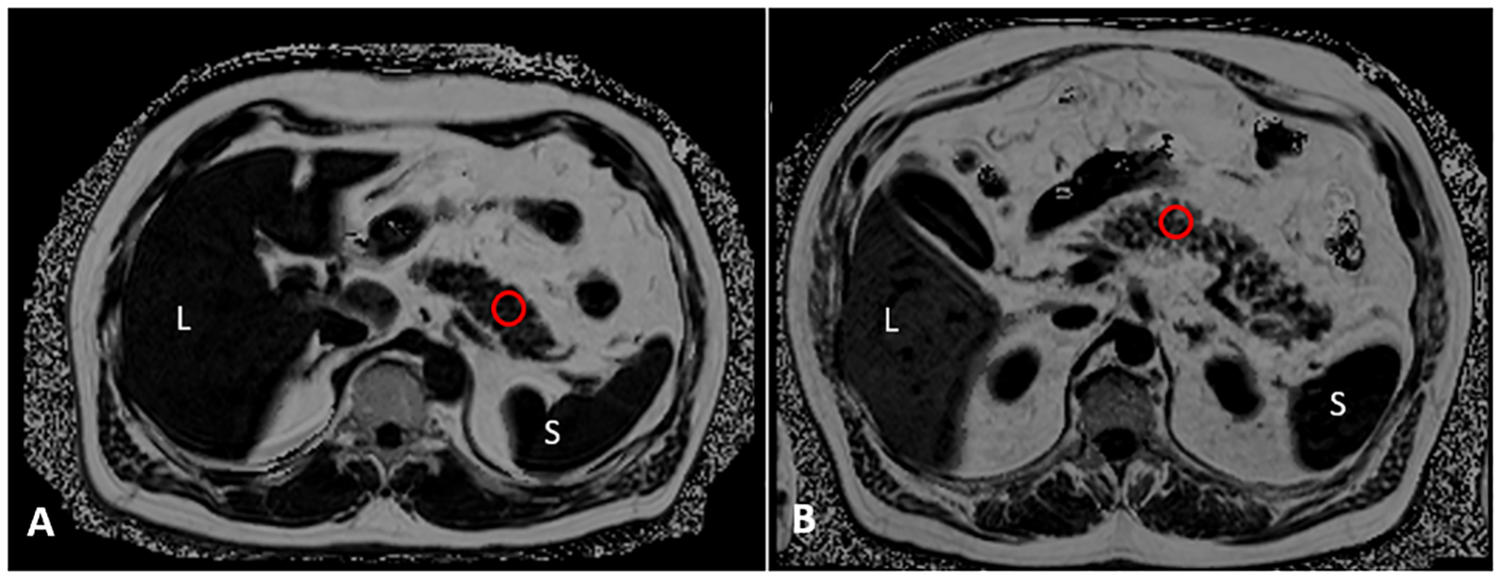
Assessment of pancreatic fat content in subjects with lower and higher pancreatic fat content undergoing 3T magnetic resonance imaging (multi-echo GRE Dixon sequence) from the general population. Pancreatic fat content was measured as proton-density fat fraction (PDFF_panc_) in a region of interest (red circle). L = liver; S = spleen.
